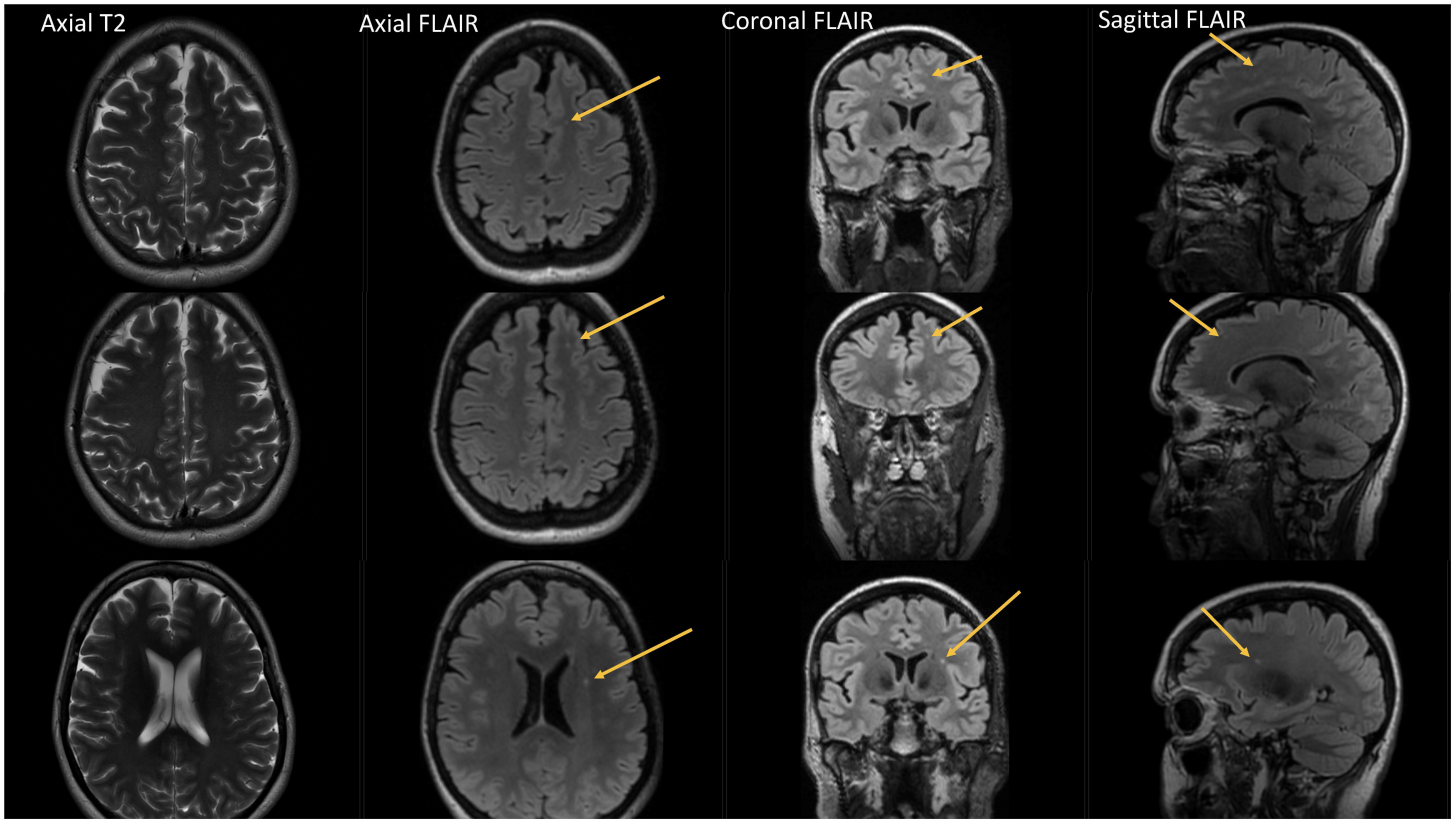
Fourty-eight year-old female with chronic migraine, no aura. No history of vascular risk factors. Axial, coronal and sagittal FLAIR images show 3 left frontal white matter lesions all of which are small and punctate in contour. Lesions are difficult to appreciate on axial T2 images.
